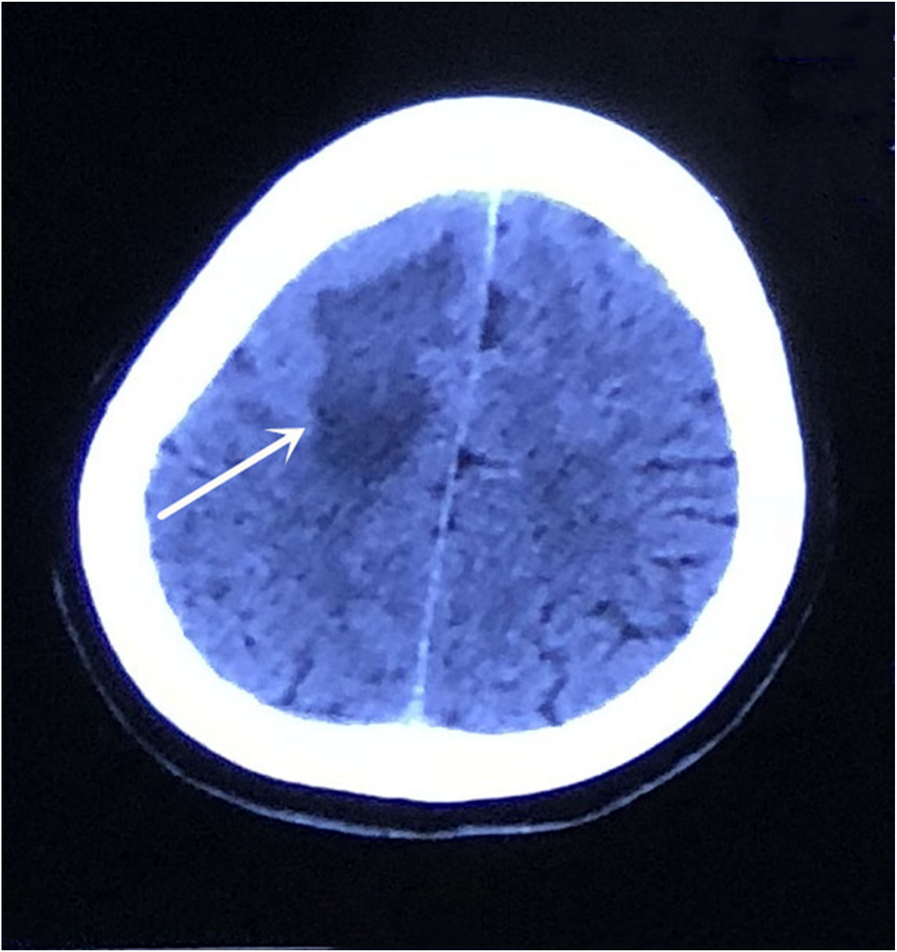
Unenhanced CT was performed at the onset of headache and showed an area of hypodensity in the right frontal lobe
